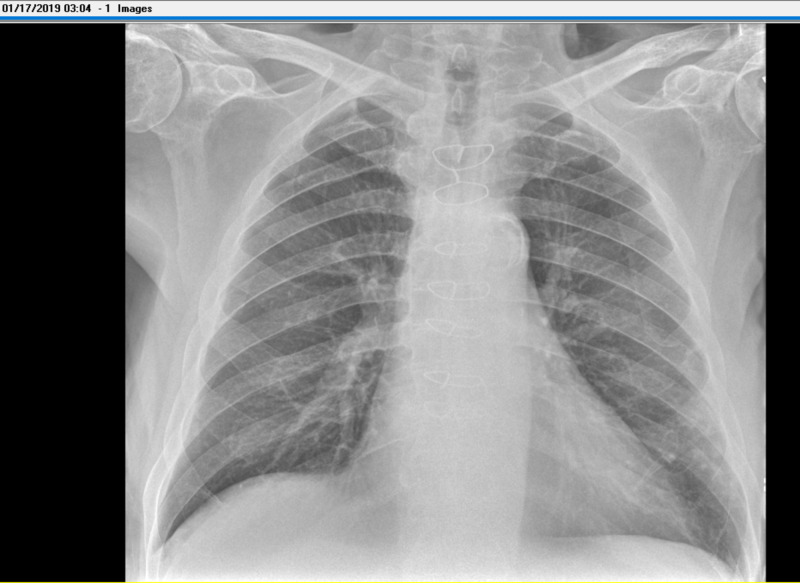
Chest X-ray Chest X-ray without acute cardiopulmonary abnormalities.
